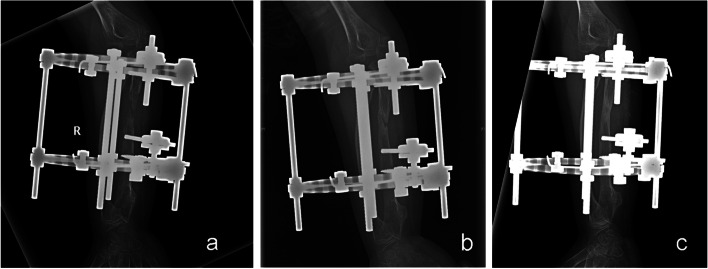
Radiographs of patient 5: a There is delayed healing, with non-union at the osteotomy site at the end of 2 months of postoperative distraction. b The non-union healed gradually with application of sustained reverse pressure over 2 months. c The distraction was re-applied for another 2 months
